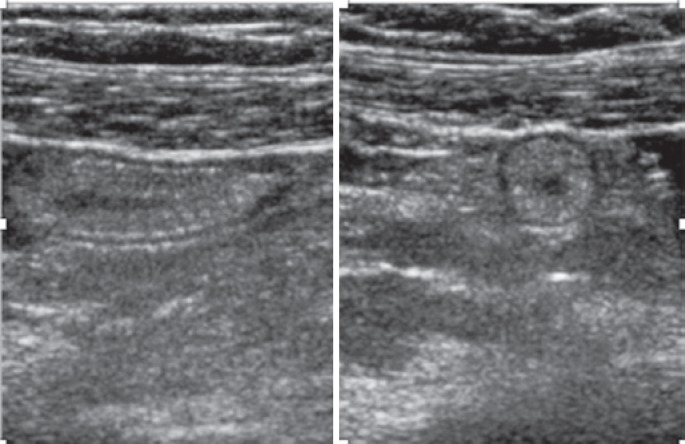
Caecal appendix with thicker wall identified in the ultrasonography
